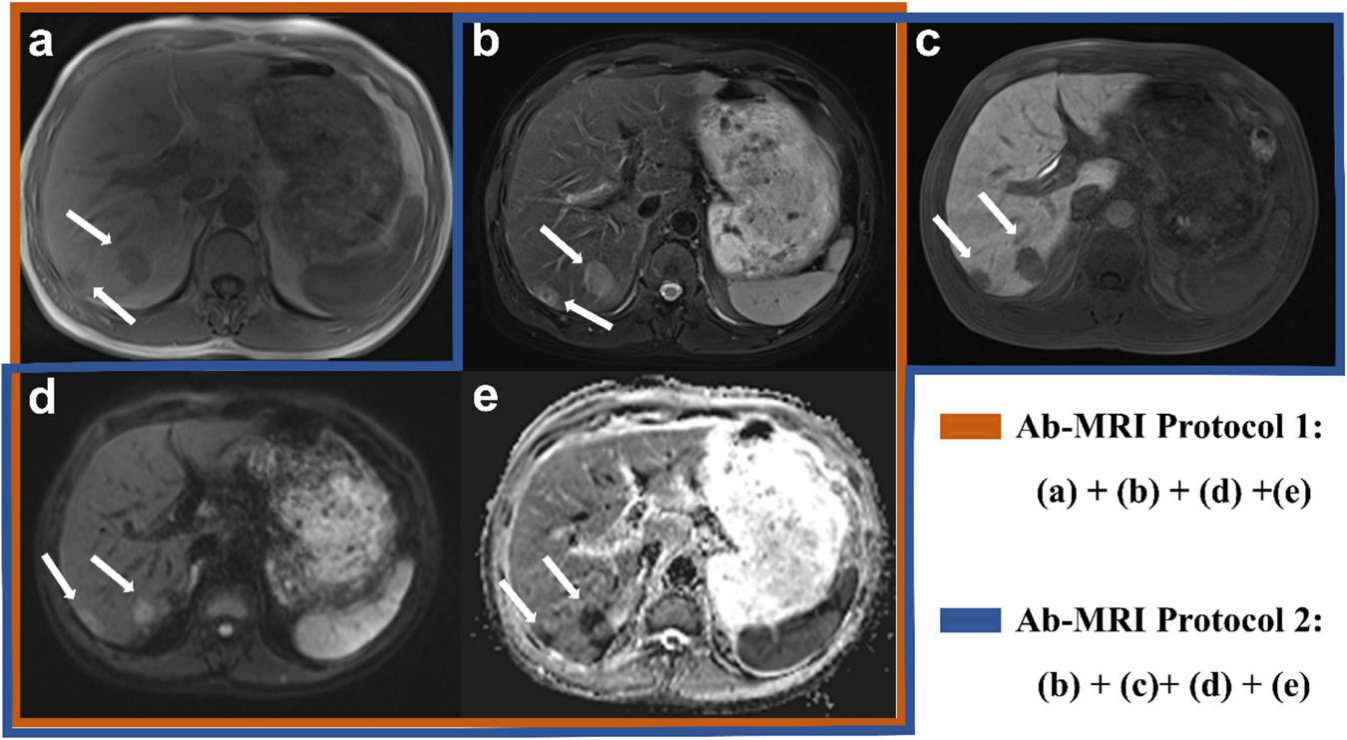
Display of two abbreviated MRI protocols in a 57-year-old male patient. a Dixon T1-weighted images (in-phase), (b) turbo spin-echo T2-weighted image, (c) hepatobiliary phase (HBP), (d) high b-value diffusion-weighted image (DWI), and (e) apparent diffusion coefficient (ADC) map. Segment VII revealed two lesions (indicated by arrows), displaying low T1 signal, high signals on DWI and T2, reduced ADC values, and notable HBP hypointensity. Consensus between two readers classified them as score 5 under both assessment protocols, correlating with a diagnosis of hepatic metastases
